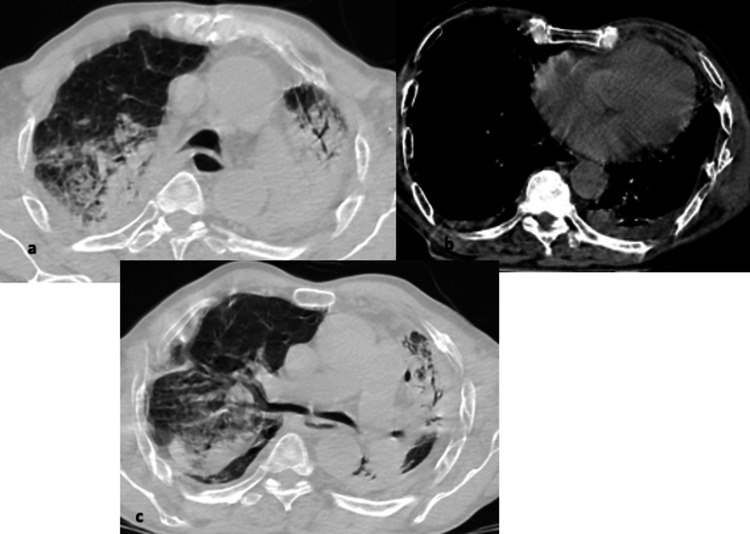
(a-c) Axial images from CT chest showing confluent consolidations bilaterally with small pleural effusions. Image quality affected by breathing and motion artifacts.
